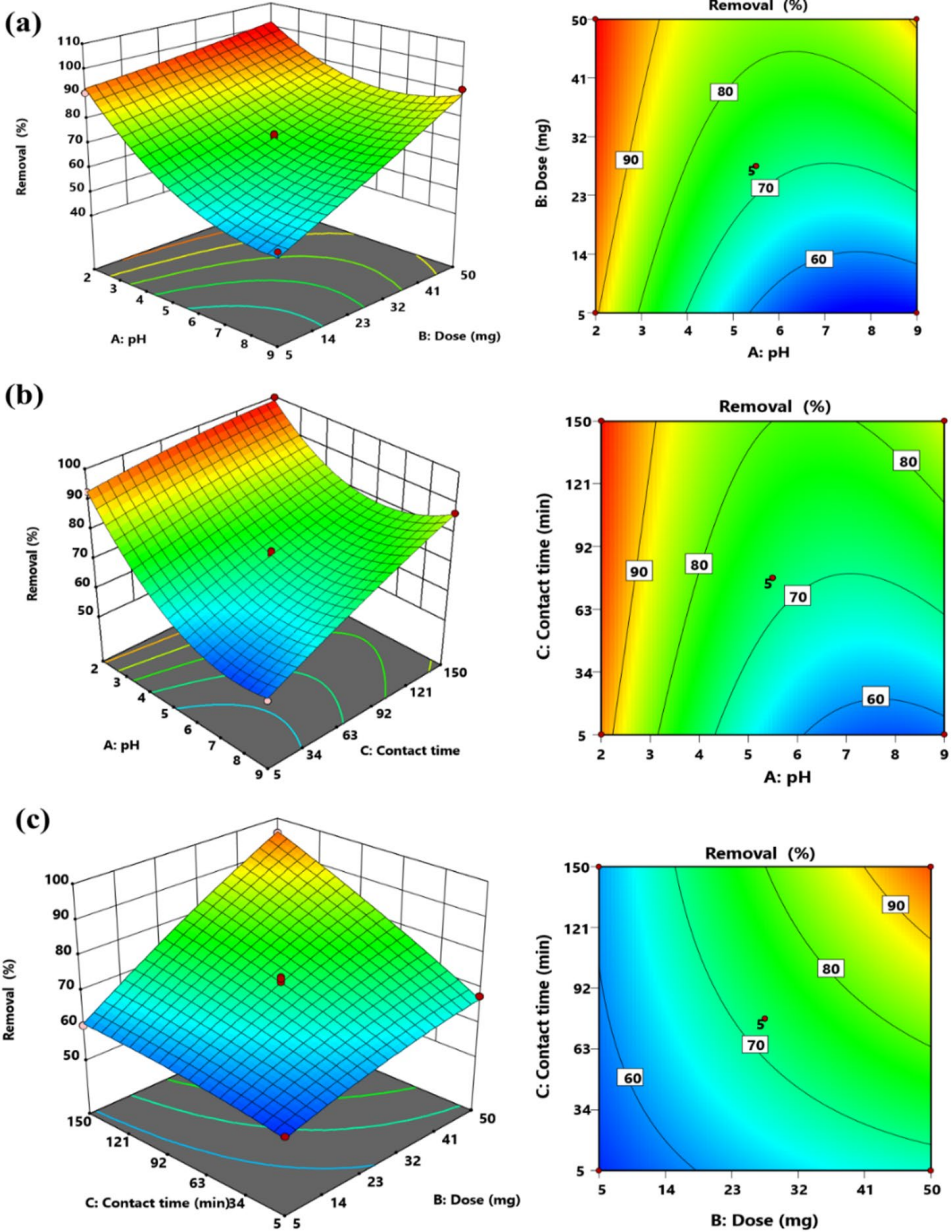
3D response surface plot showing the interaction between (a) initial MO mass versus pH, (b) contact time versus pH, (c) CT versus mass for MO removal.
